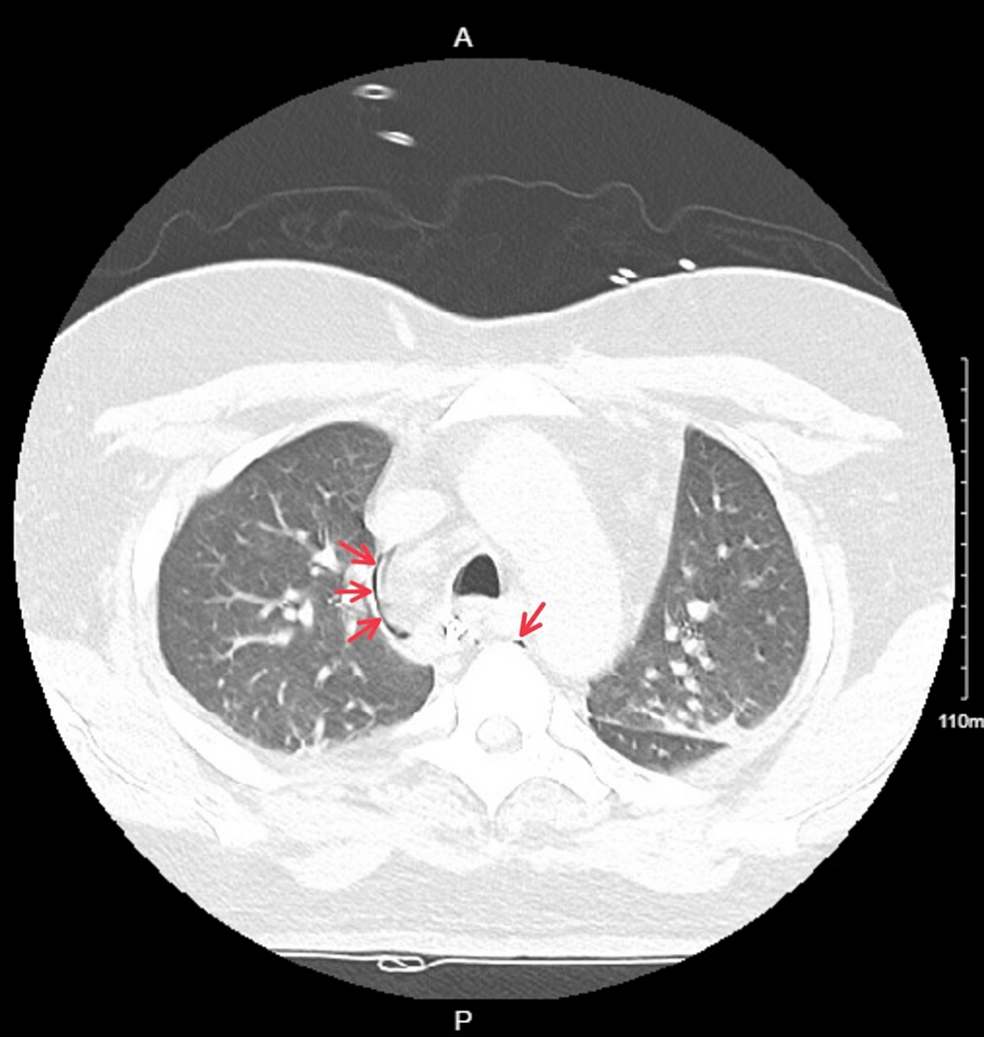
CT chest showing pneumomediastinum Air in the mediastinum can be seen as dark spaces (marked by red arrows).
